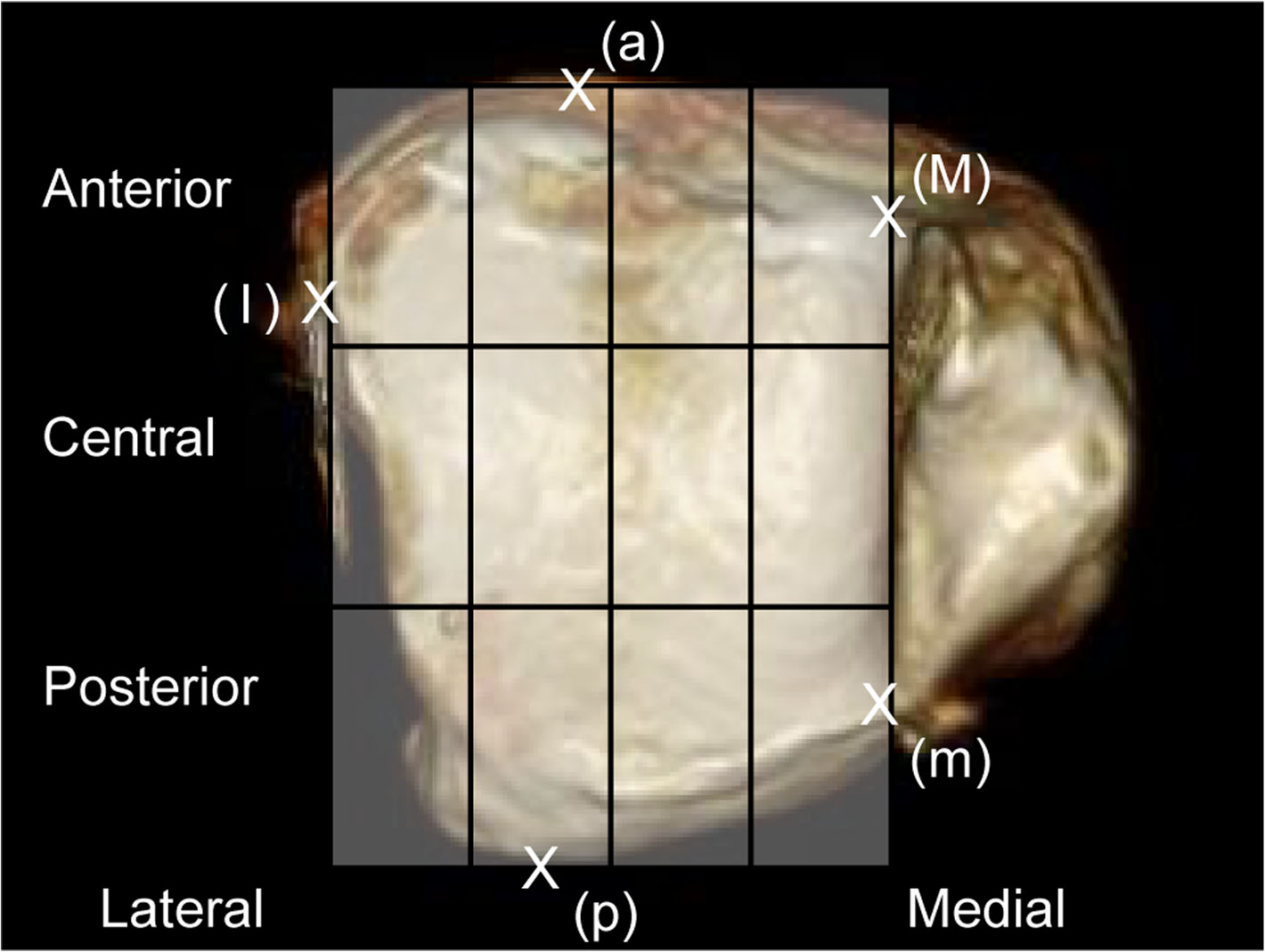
Distal articular surface three-dimensional computed tomography images of the tibia. Assessment was performed using a rectangle connecting the line Mm, anterior border (a), posterior border (p), and lateral border (l). The rectangle was divided into three sections (anterior, central, and posterior) anteroposteriorly and four sections mediolaterally
